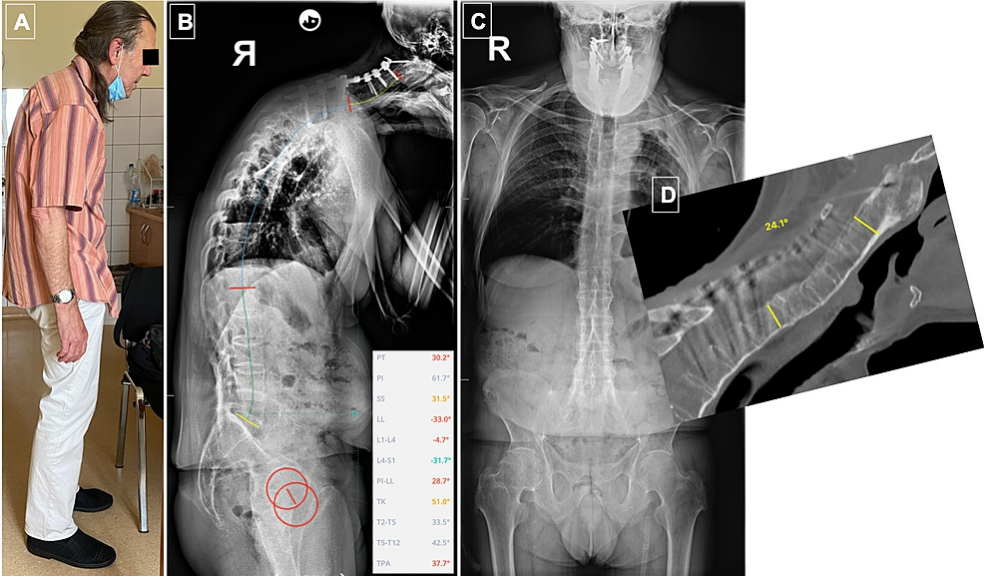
Six months after surgery A) Clinical picture showing satisfactory head position; B) Full spine anteroposterior (AP) and lateral X-rays; C) Significantly unbalanced spine sagittally, significantly positive sagittal vertical alignment (SVA); D) CT scan showing fusion of the anterior column of the spine
